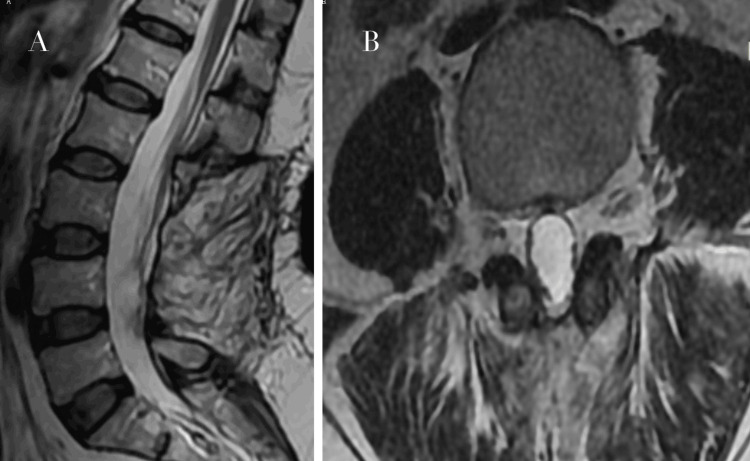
Postoperative lumbar MRI demonstrating complete tumor resection (A) Sagittal view showing restoration of cerebrospinal fluid signal and decompression of the thecal sac without residual mass. (B) Axial view confirming absence of residual tumor and adequate decompression of the spinal canal.
